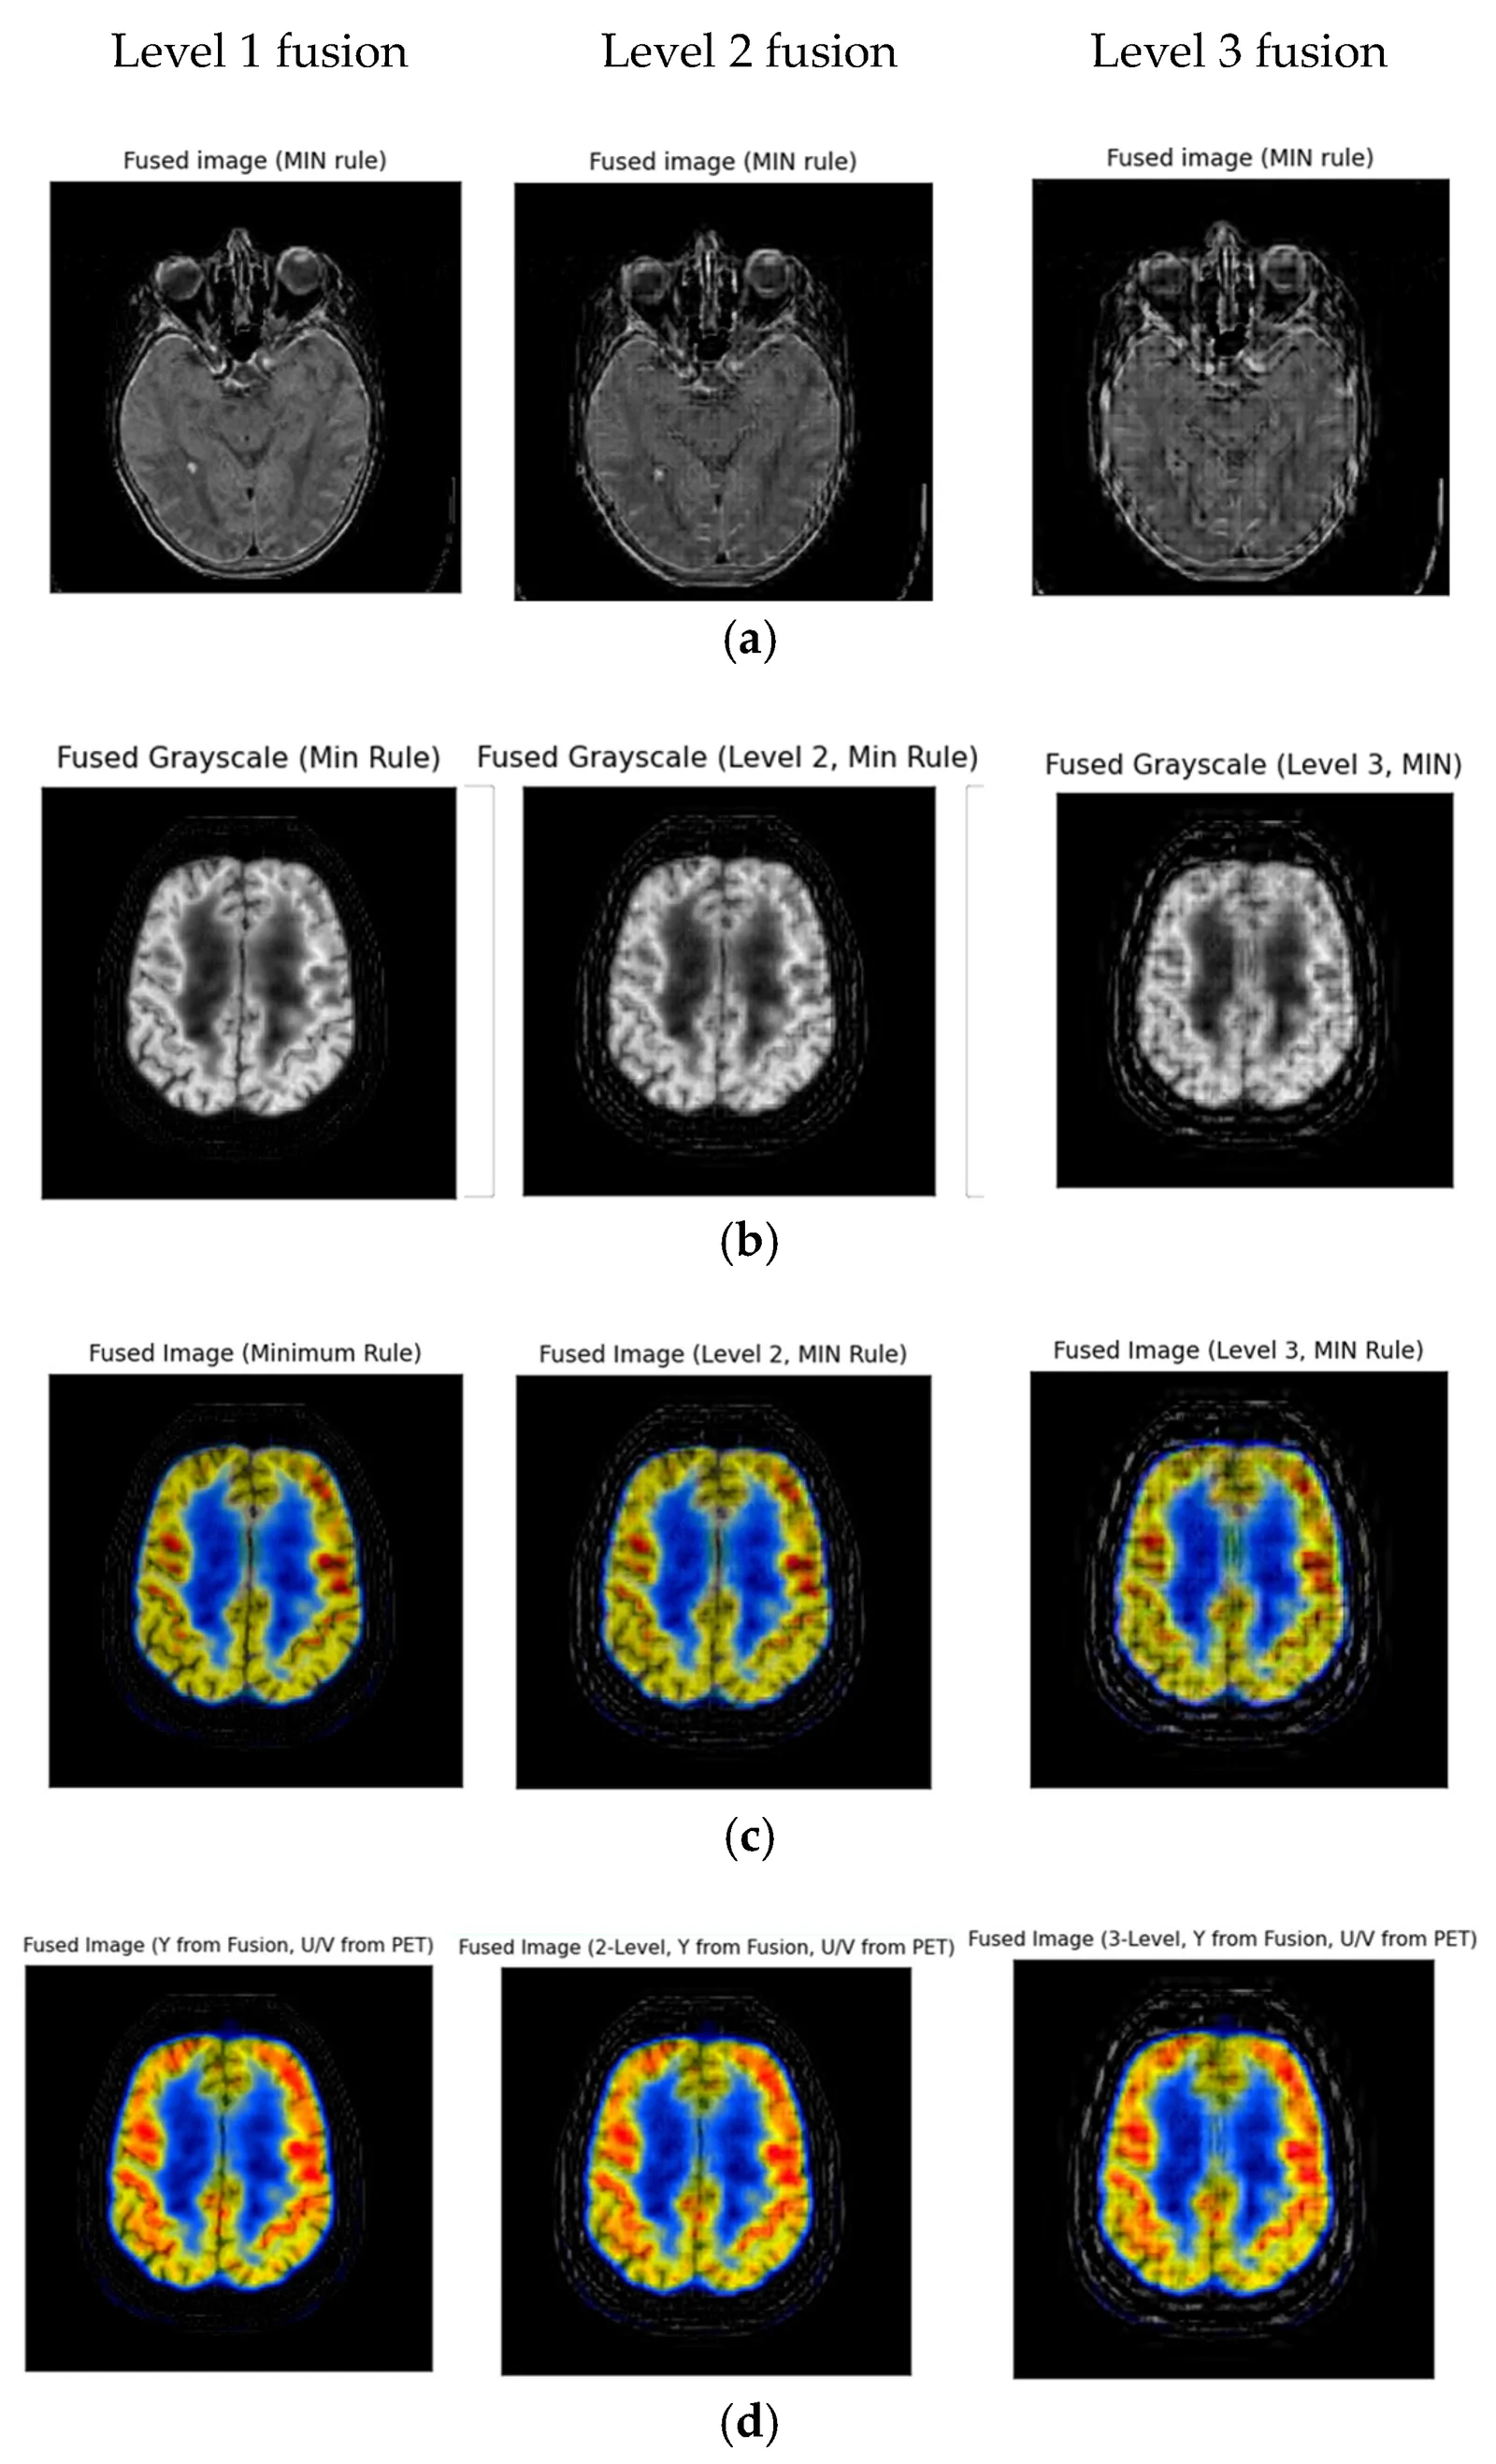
Comparison of one-, two-, and three-level fusion results, using the minimum fusion rule with the ‘bior4.4’ wavelet: (a) CT and MRI; (b) PET grayscale and MRI; (c) PET without conversion and MRI; (d) PET YUV conversion and MRI.
